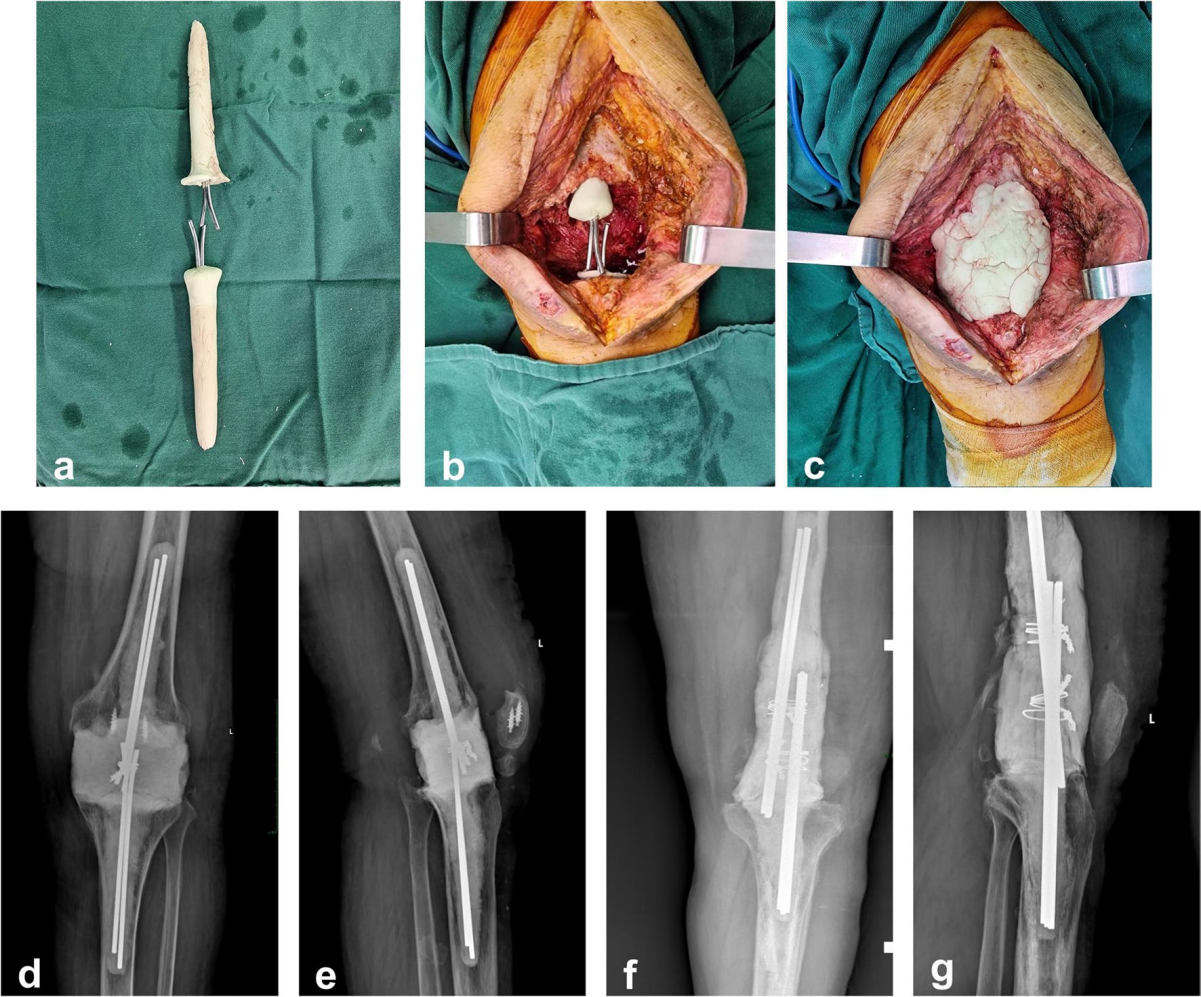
a. Self-constructed intramedullary cemented rods. b intramedullary cement rods inserted tightly into the femur and tibia. c The gap was filled with cement. d, e A 79-year-old woman was diagnosed as chronic knee periprosthetic joint infections, her past medical history is positive for diabetes and rheumatoid arthritis. She had undergone twice debridement, presented with sinus tract and knee extensor failure. Static and permanent cement spacers was implanted. Anteroposterior and Lateral view of Radiograph of the spacer at 2.4-year follow-up. f, g An 80-year-old male was diagnosed as chronic knee periprosthetic joint infections, his past medical history is positive for rheumatoid arthritis and atrial fibrillation. There was sever bone defect during the second debridement. Static and permanent cement spacers was implanted. Anteroposterior and Lateral view of radiograph of the spacer at 2-year follow-up
